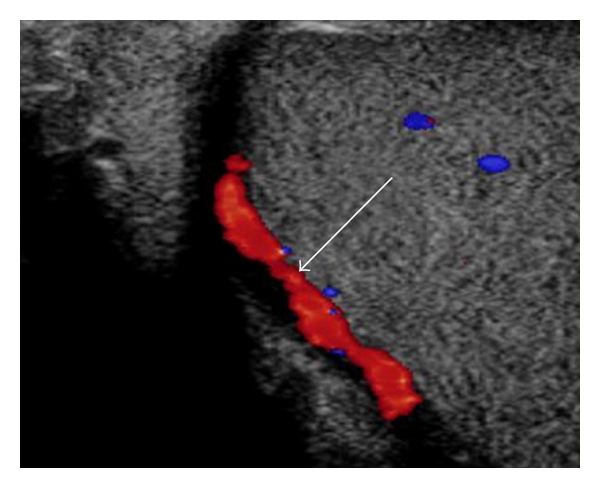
Ultrasonogram of a testis using color pulsed-wave Doppler ultrasonography showing blood flow within the marginal artery (arrow).
